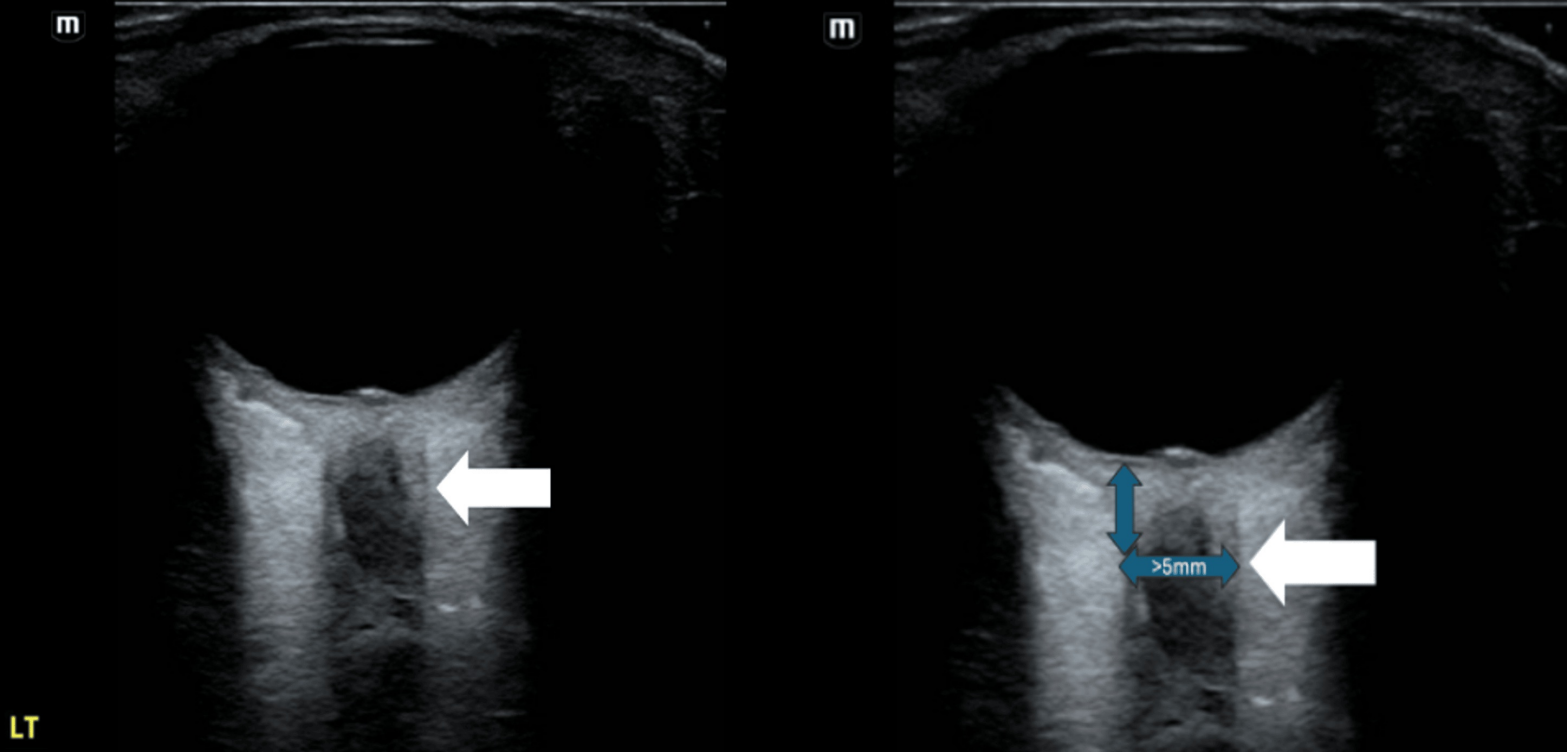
Bedside ocular ultrasound of the left eye demonstrating an abnormal optic nerve consistent with optic neuritis (white arrow).
